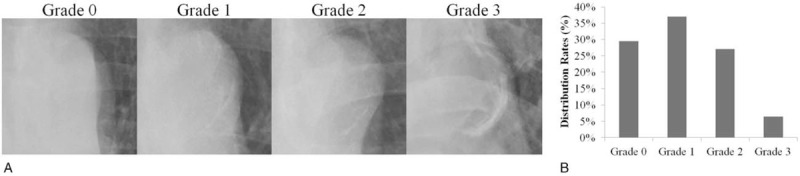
AAC extent across a 4-point scale and distribution of AAC grades. (A) The extent of AAC detected by chest x-ray was divided into 4 grades: Grade 0, no visible calcification (panel A); Grade 1, small spots of calcification or a single thin area of calcification of the aortic knob (panel B); Grade 2, one or more areas of thick Calcification (panel  C); Grade 3, circular calcification of the aortic knob (panel D). (B) Distribution of AAC grades in all patients. AAC = aortic arch calcification.
